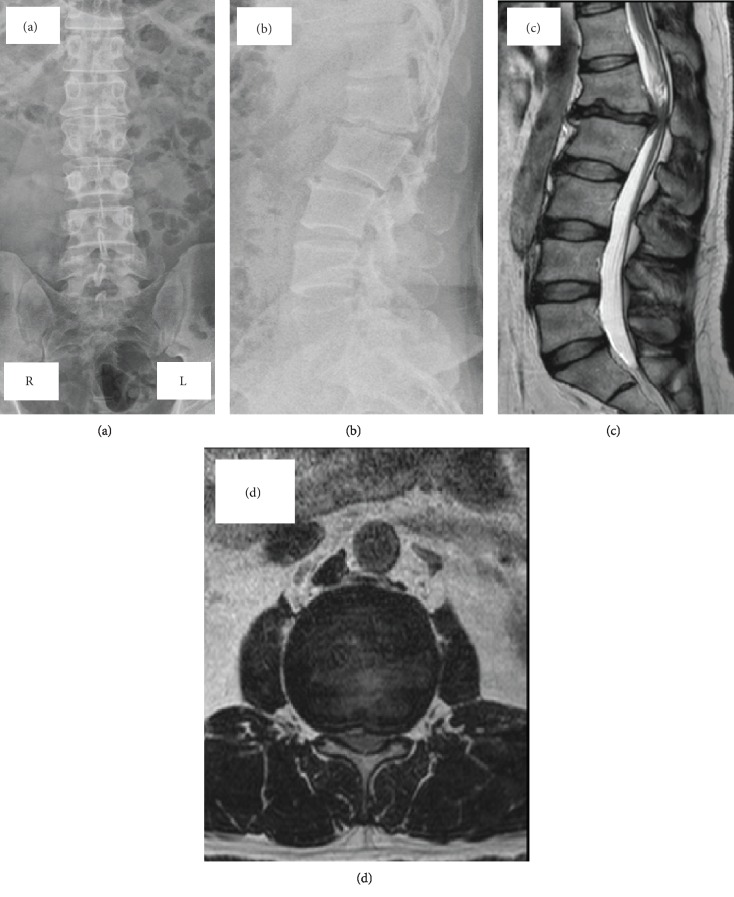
Anterior-posterior radiograph (a) and lateral radiographs (b) of the lumbar spine showing severe degenerative change at L1-2 and compression of the L1 vertebral body. Sagittal (c) and axial (d) T2-weighted magnetic resonance images showing degeneration of the disc and severe canal stenosis at the L1-2 level. R indicates right side; L, left side.
